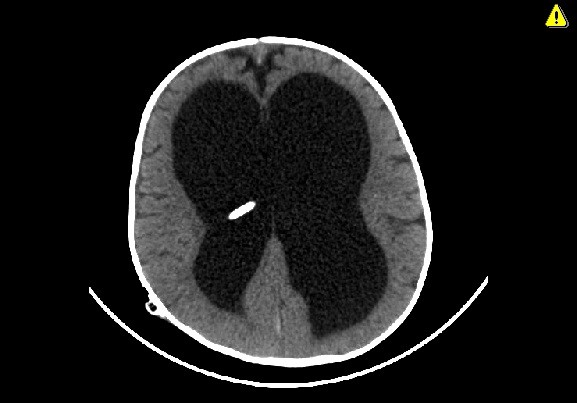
Axial computed tomography scan revealing no supratentorial intraparenchymal or intraventricular hemorrhage related to the catheter insertion.
